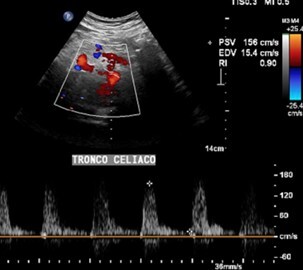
Color Doppler ultrasound shows a patent celiac trunk without hemodynamically significant stenosis, with low-resistance flow and normal velocities (155 cm/s; aortic ratio, 1.9).
